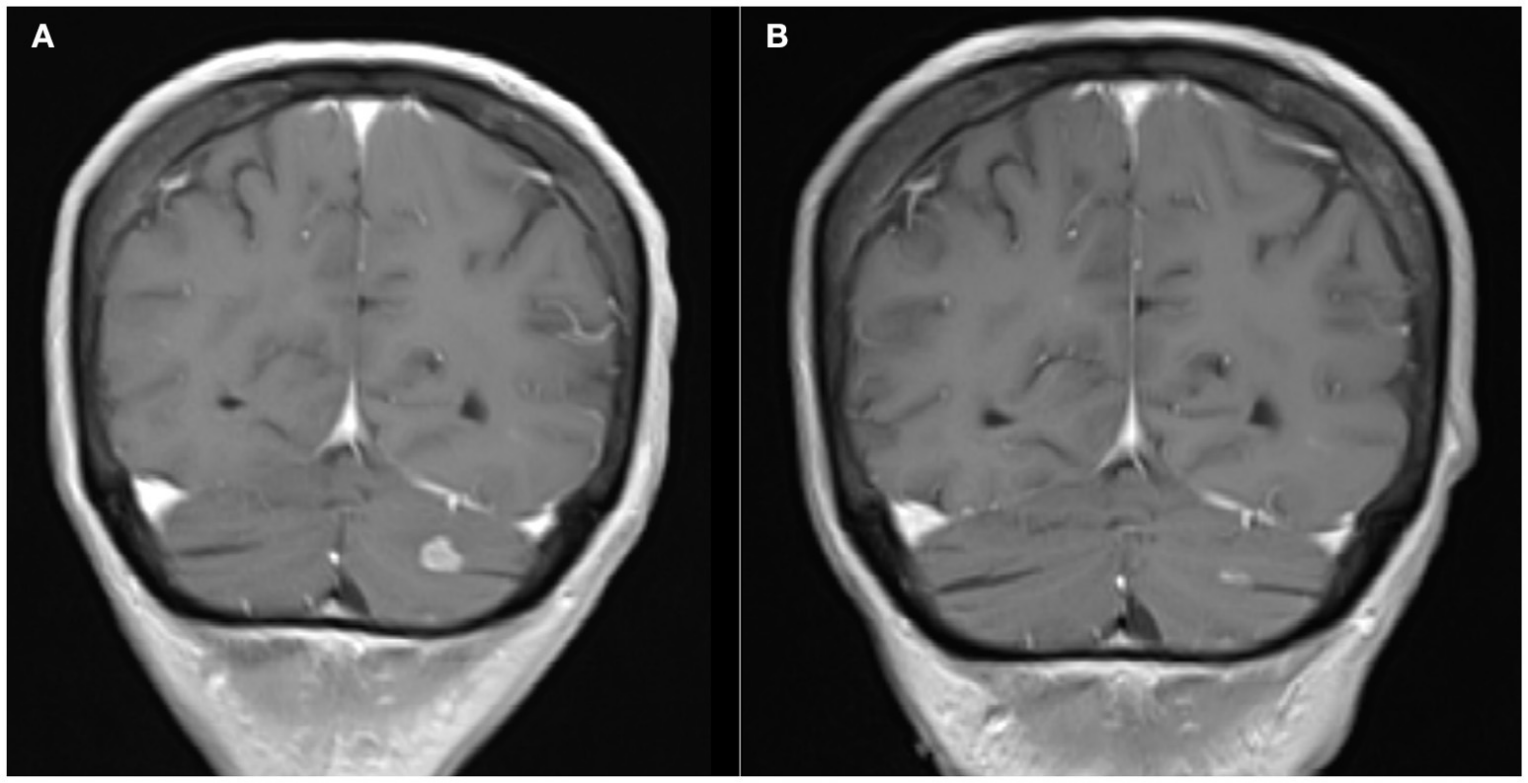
(A) Coronal Brain MRI, T1 with contrast showing a hyperintense lesion in the left cerebellar lobe before systemic therapy with TDM1. (B) Coronal Brain MRI, T1 with contrast showing decrease in the size of the lesion after treatment.
